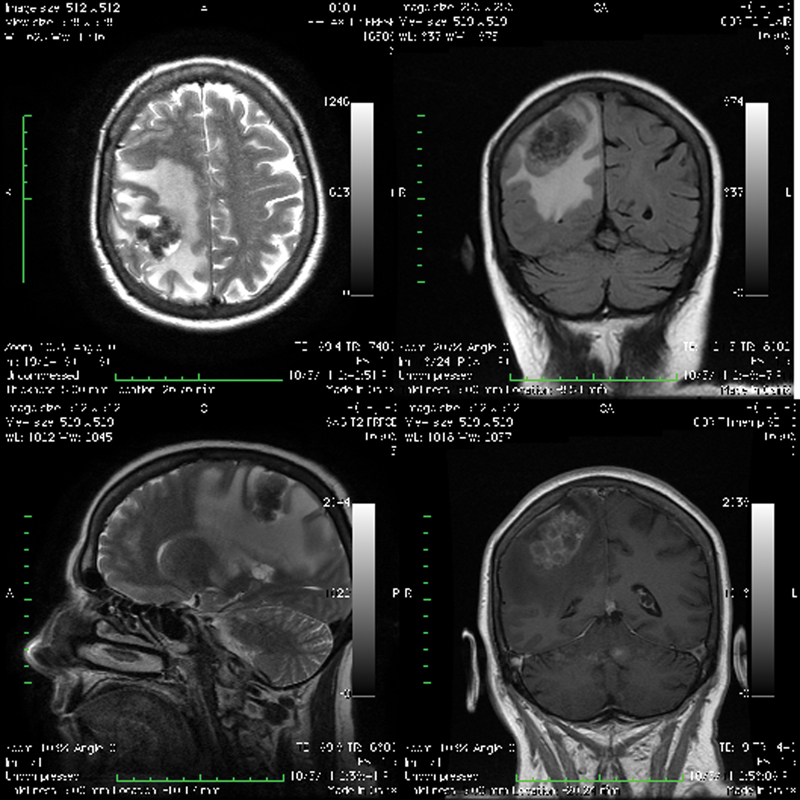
Brain magnetic resonance imaging. Axial view T2-weighted image (upper left) and coronal view T2-weighted image (bottom left) showing the irregular hypo-/hyperintense nodular lesion in the right postcentral gyrus with an extensive perifocal edema. Coronal fluid-attenuated inversion recovery image (upper right) showing the nodular iso-/hypointense lesion with a massive perifocal edema in the right parietal lobe. Coronal T1-weighted image with contrast enhancement (bottom right) showing the multilocular cystic lesion with a perifocal edema resembling a bunch of grapes.
